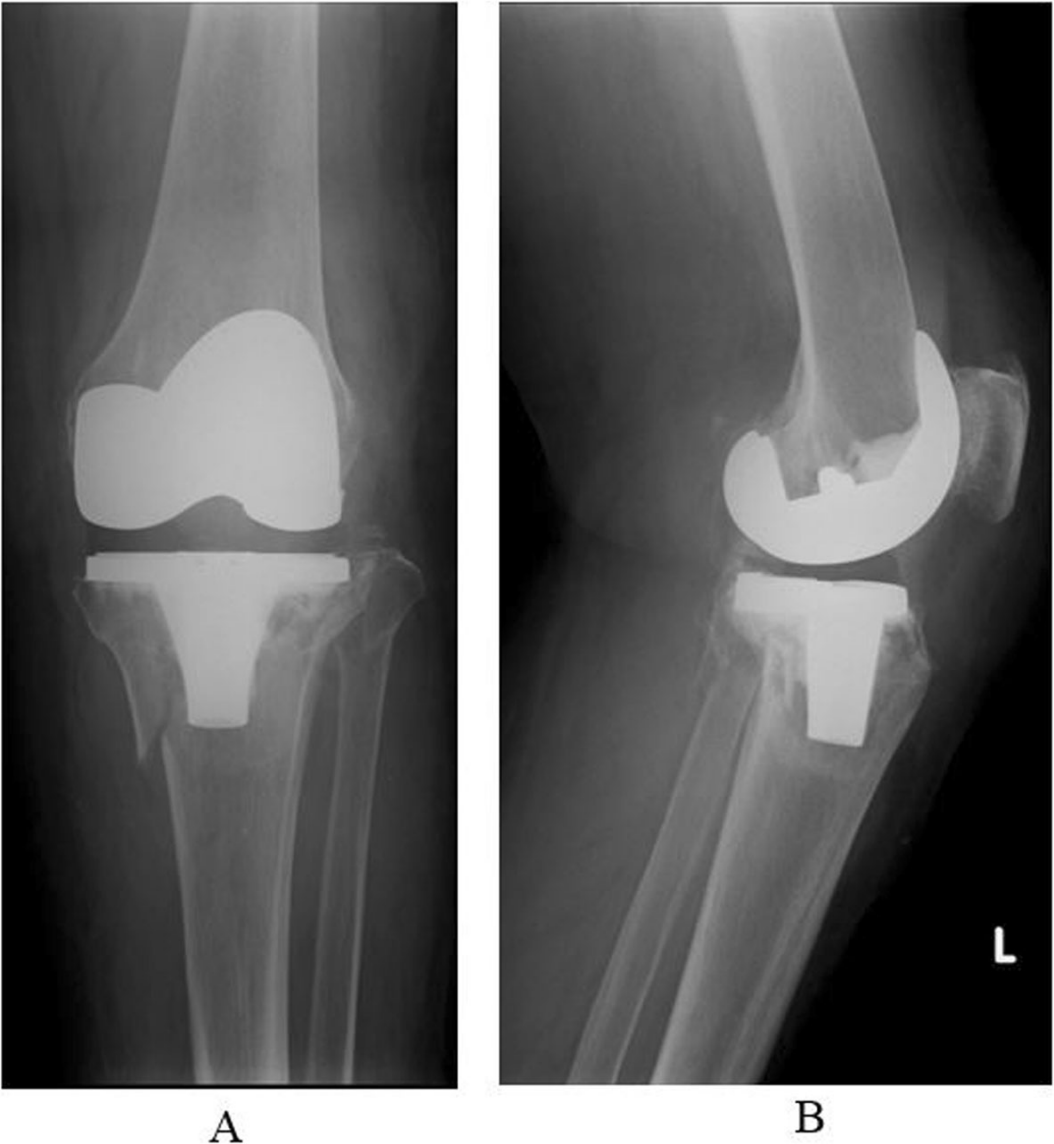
a Preoperative antero-posterior X-ray. b Preoperative lateral angle X-ray
